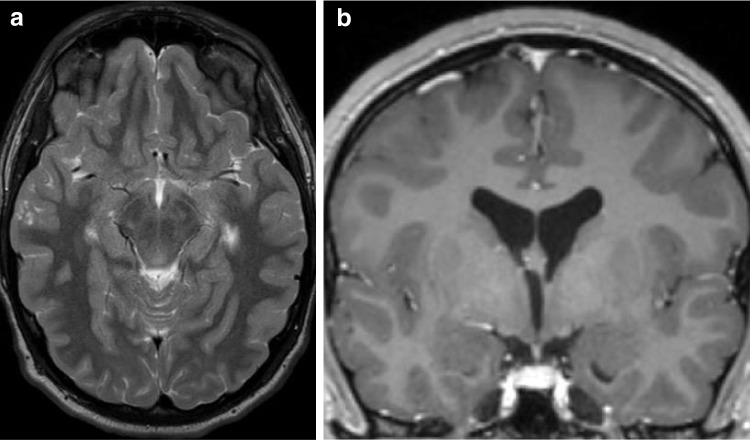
Multinodular and vacuolating neuronal tumor (MVNT) in a 15-year-old male who presented with a seizure. Axial T2-weighted (a) and coronal T1-weighted contrast-enhanced (b) MR images reveal tiny, clustered, ovoid, T2-hyperintense, non-enhancing foci located along the deep aspect of the cortical ribbon of the right temporal lobe
